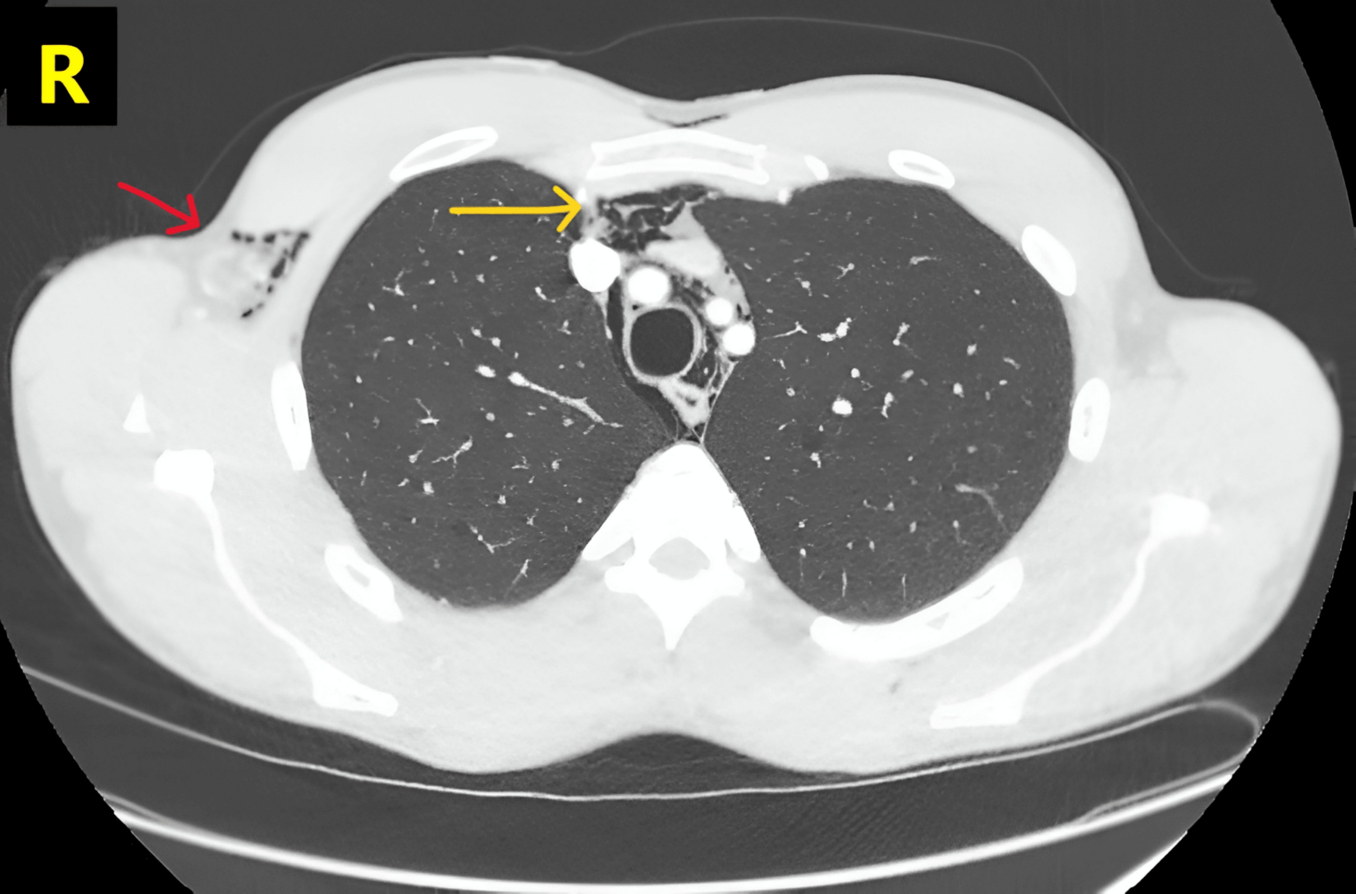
CT of the thorax (shown on lung window) showing pneumomediastinum in the superior mediastinum (yellow arrow), with additional subcutaneous emphysema visible inferolateral to pectoralis major on the right side (red arrow).
